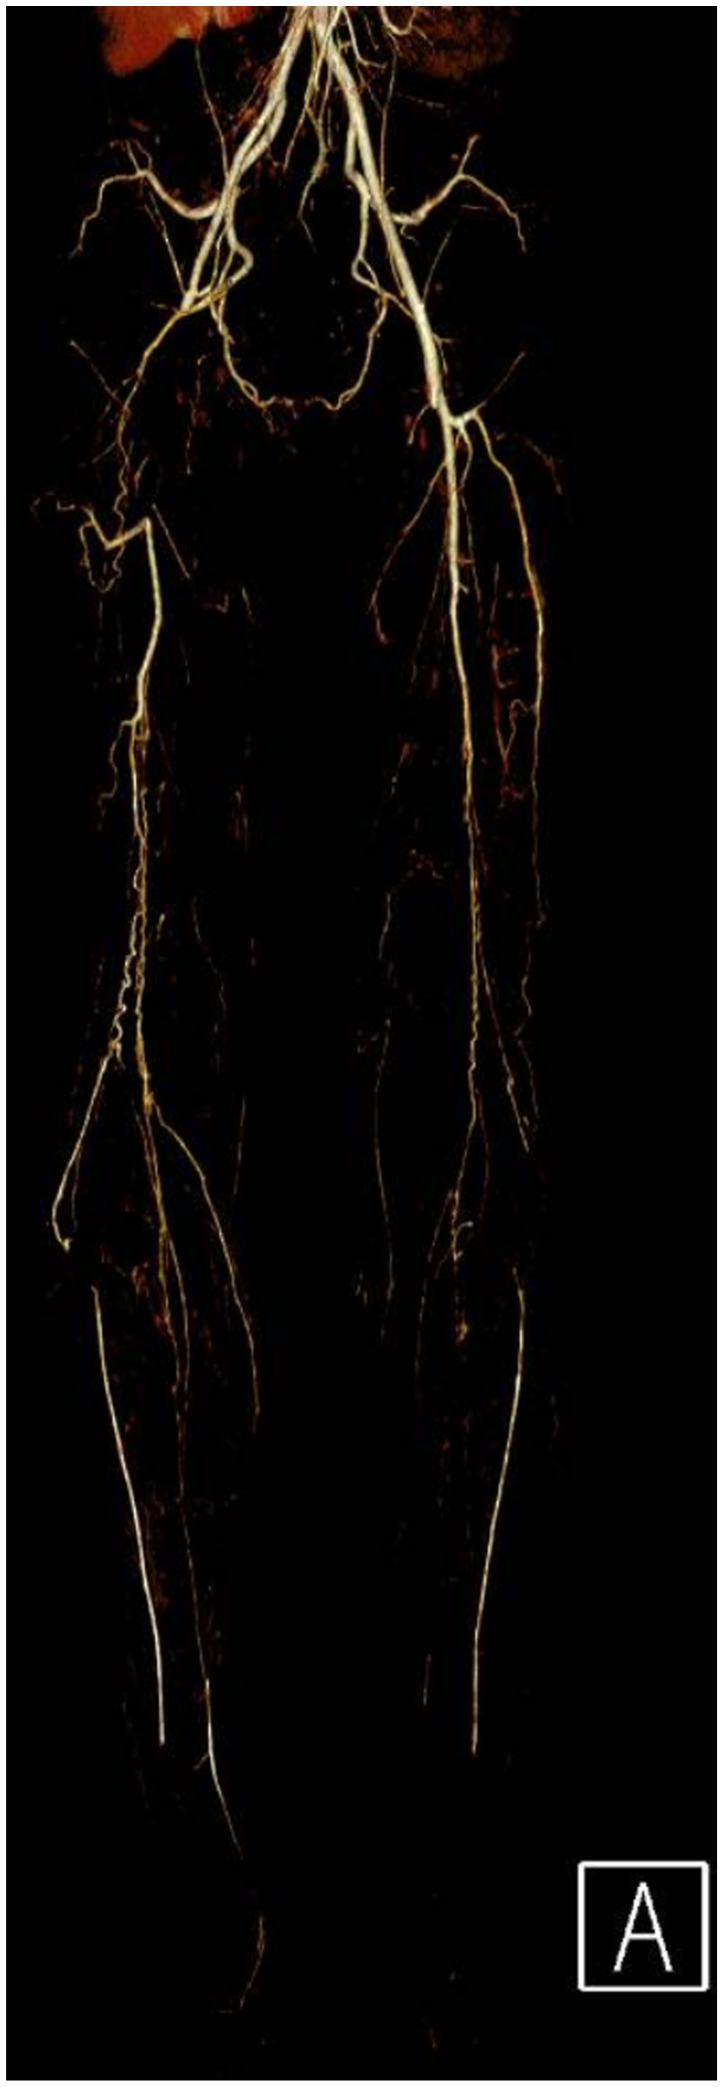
Three-dimensional reconstruction of the patient’s computed tomography angiography with bilateral lower extremity runoff. Note the right superficial femoral artery occlusion with distal reconstitution of the anterior and posterior tibial arteries.
